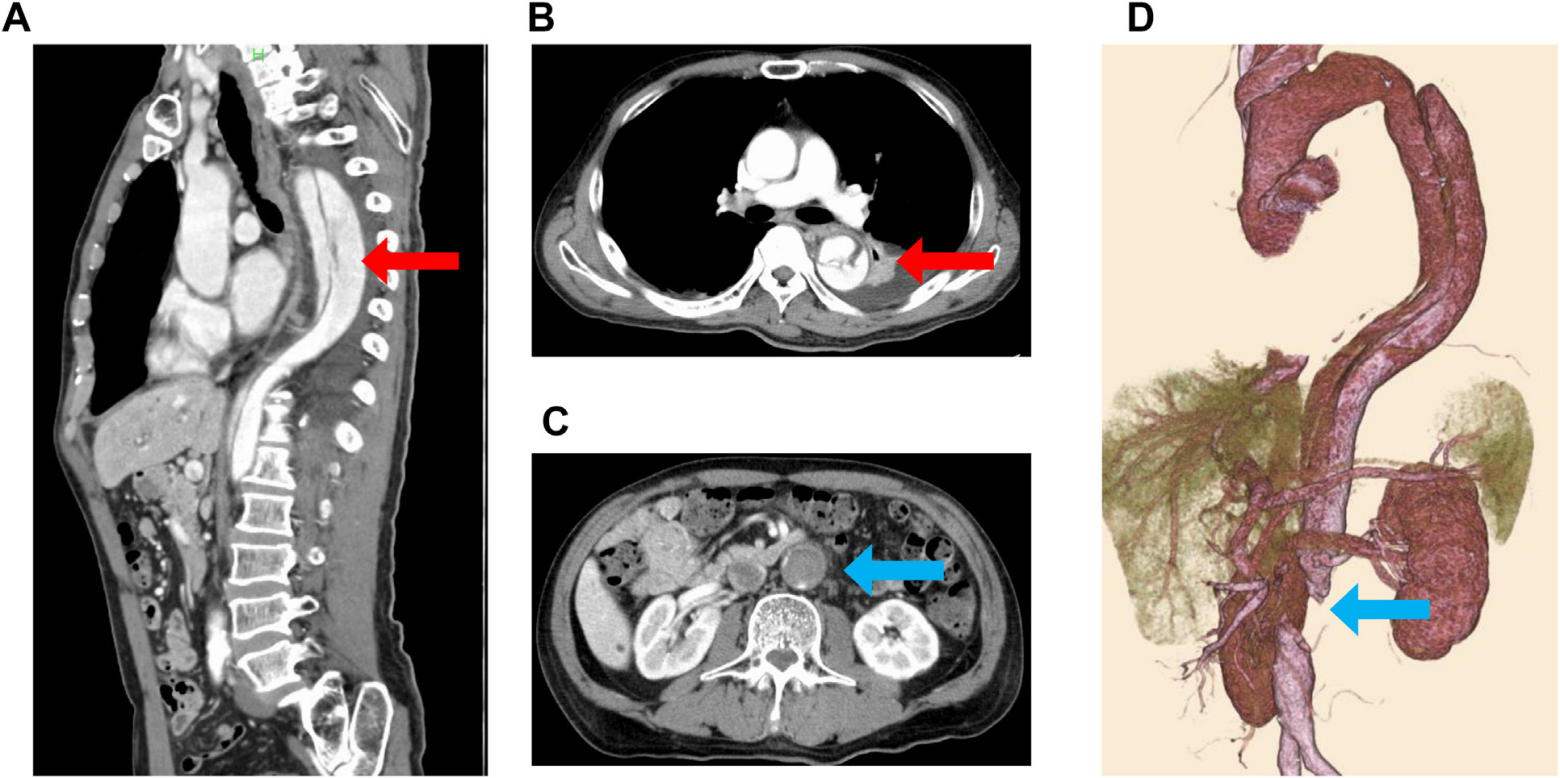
Three-dimensional reconstruction of contrast-enhanced computed tomography images in mid-sagittal view (A), axial view at the proximal descending aorta level (B), and axial view at infrarenal abdominal aorta level (C). *Red arrows* indicate an entry tear; and *blue arrows,* an occluded region of the abdominal aorta.
